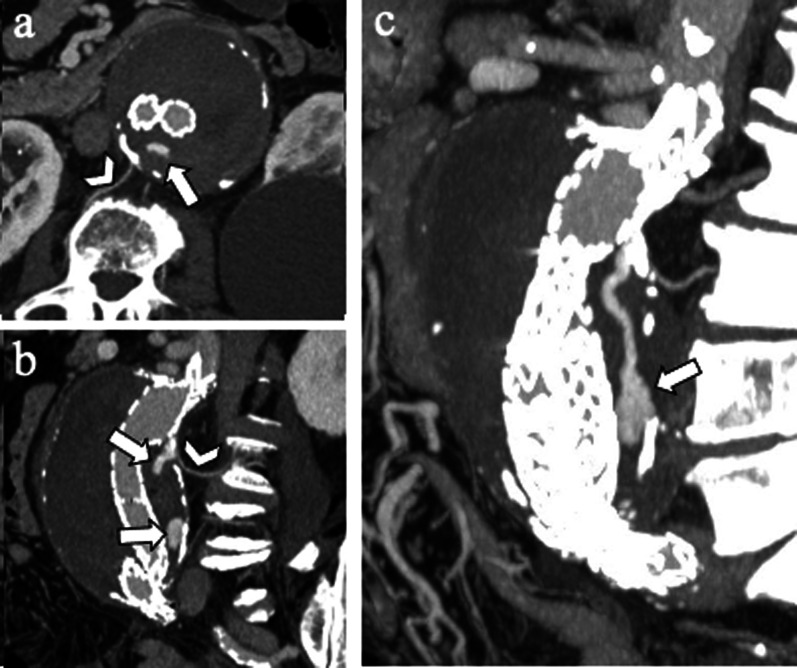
86-year-old man with *type IIa* endoleak. a Axial and (b, c) sagittal maximum intensity projection CT angiography images show *type II* endoleak (arrows) due to retrograde perfusion of a lumbar artery (arrowheads)
